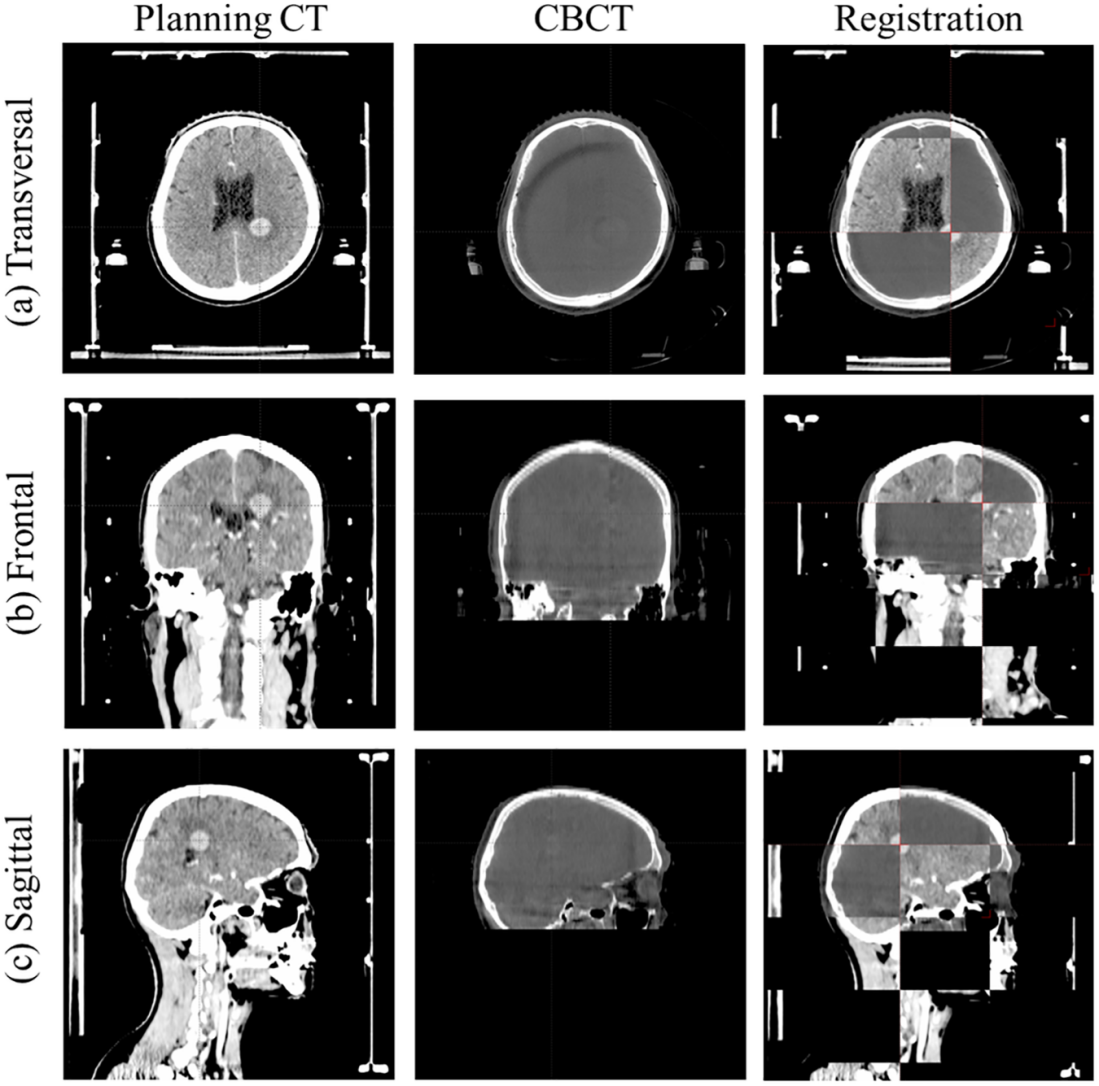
Image registration using planning computed tomography (CT) and cone-beam computed tomography (CBCT) for patient #18 with offline 6D fusion in offline review. (a) Axial planes; (b) Coronal planes; and (c) Sagittal planes.
